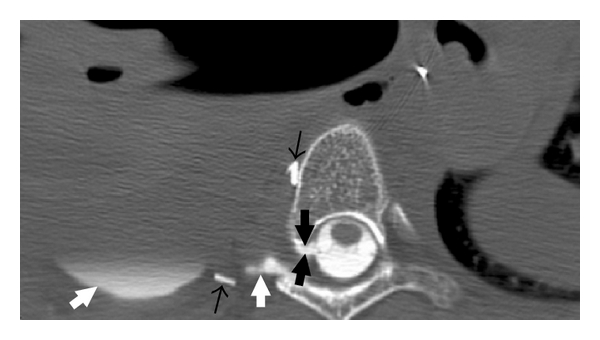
Axial CT obtained after contrast myelogram demonstrates the subarachnoid-pleural fistula (thick black arrows) establishing communication between the spinal canal and the right pleural effusion. Contrast is observed to layer dependently in the right pleural space (white arrows). Note the adjacent surgical clips (thin black arrows).
